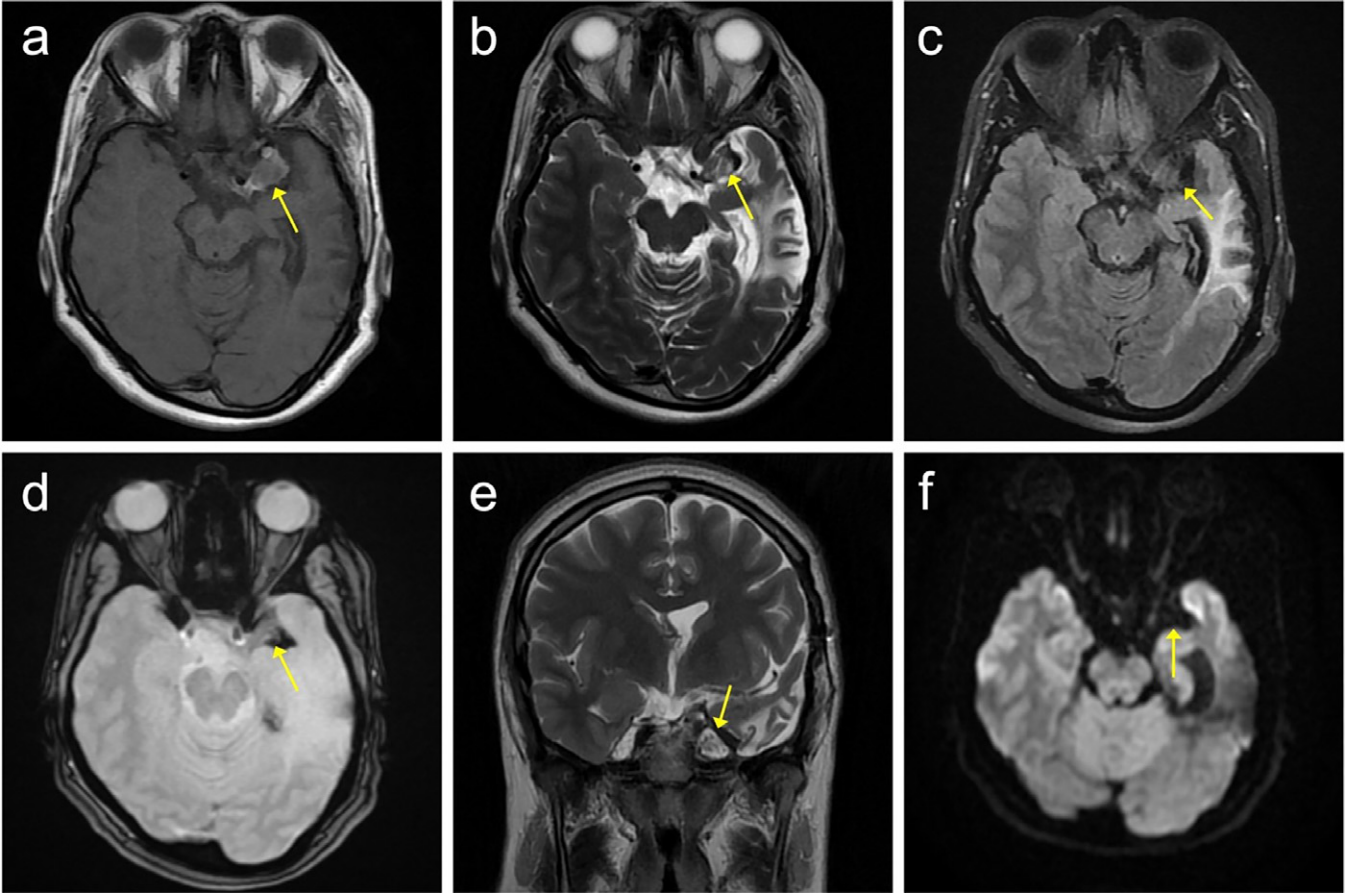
Follow-up Brain MRI performed 1 year after surgery. (A) Axial T1W (B) Axial T2W (C) Axial T2 FLAIR (D) Axial T2 GRE (E) Coronal T2W (F) DWI. A high T1 and low T2 signal intensity lesion is observed in the left middle cranial fossa, without enhancement, suggesting a possible remnant of calcification from a previous tumor. The remnant tumor is indicated by the yellow arrow in all sequences.
